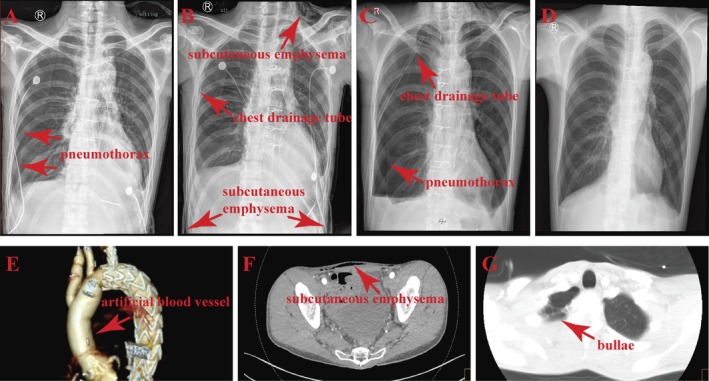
Computed tomography angiography images and chest radiographs. (A–C, F) Imaging of pneumothorax and cervical and abdominal SE (arrow). (D) SE had been almost completely absorbed at the time of discharge. (E) Three‐dimensional reconstruction of the ascending aortic graft (arrow). (G) Right apical pulmonary bullae (arrow).
